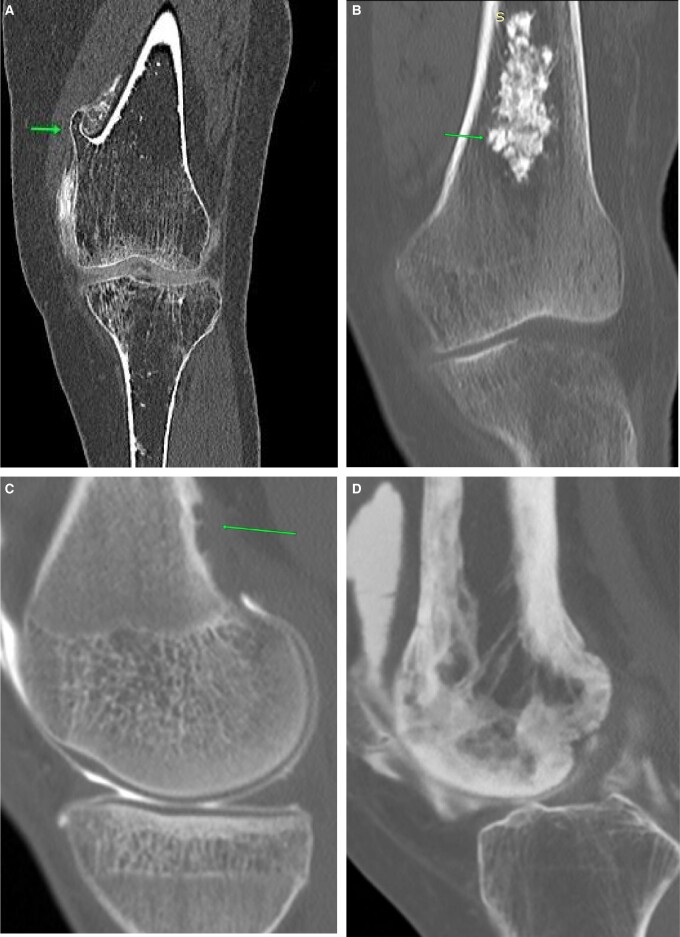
Bony pathology: (A) classical osteochondroma (arrow). (B) Classical enchondroma (arrow) in non-referred opposite knee picked up on CT scanogram and subsequently imaged. (C) Incidental fibrous cortical defect (arrow). (D) Classic Paget’s disease of femur.
